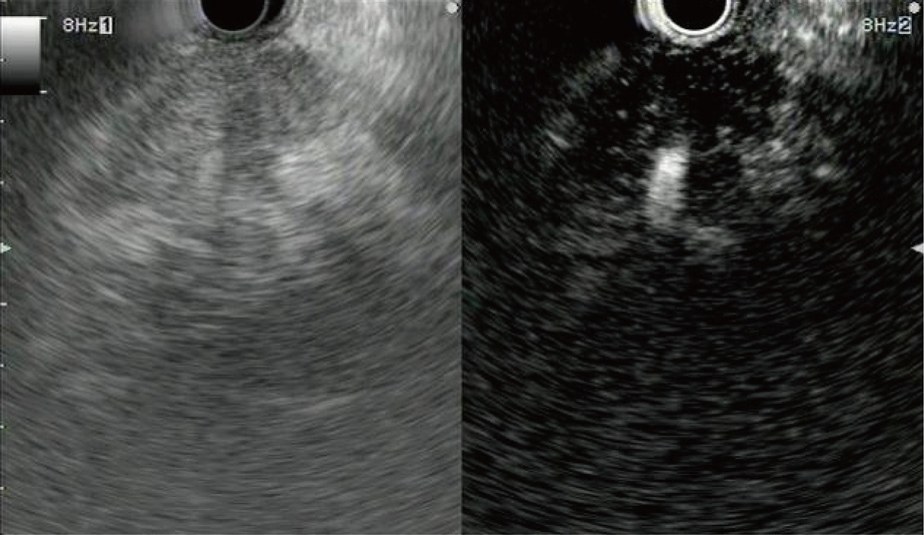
Representative example of a pancreatic adenocarcinoma with hypoenhancement. Conventional endoscopic ultrasonography (left) shows a heterogeneous hypoechoic area without a clear margin at the pancreas head. Contrast-enhanced harmonic endoscopic ultrasonography (right) indicates that most of the area is hypovascular and the remaining area is hypervascular compared to the surrounding tissue. An irregular margin is visible.
